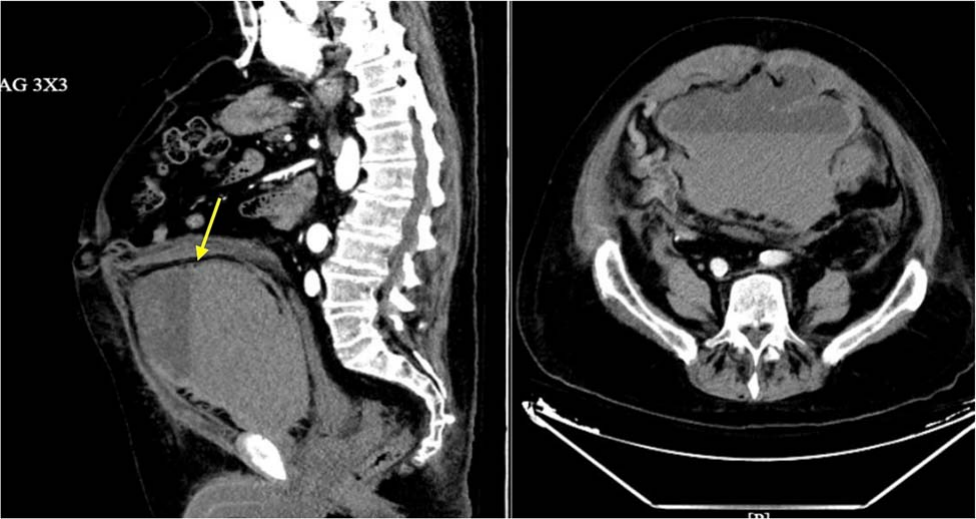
CT angiography of the abdomen and pelvis: Contrast-enhanced CT showing a large, complex hematoma (arrow) arising from the rectus sheath in the anterior pelvis, measuring approximately 14.0 × 19.0 × 15.9 cm. Subtle contrast layering in the delayed venous phase suggests mild active venous bleeding.
